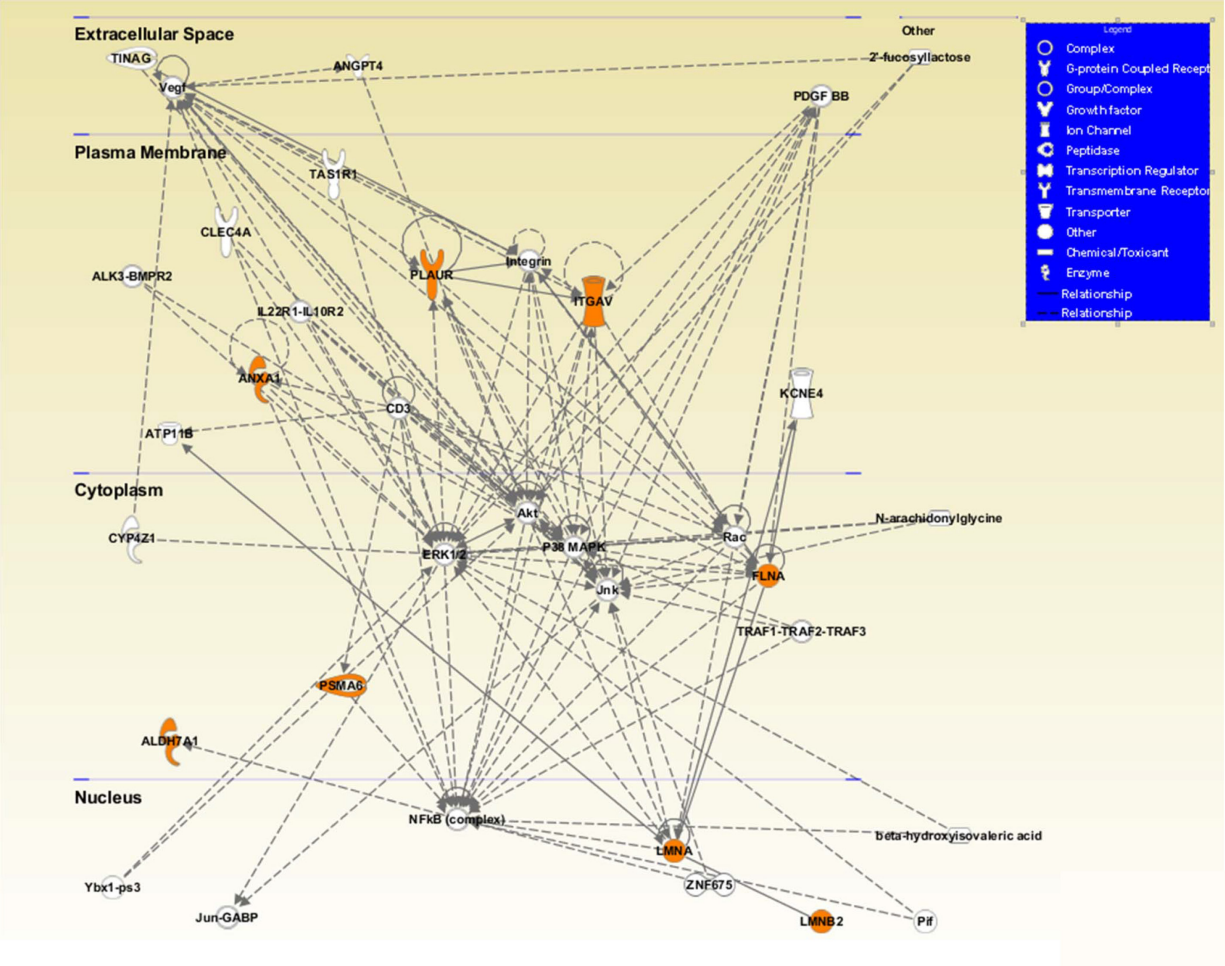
Visual representation of the principal network generated by Ingenuity Pathway Analysis (IPA) The network included 6 of the 7 validated proteins with direct and indirect relations (ANXA1, FLMNA, PSMA6, ALDH7A1, LMNA, LMNB2) connected with both αv (ITGAV) and uPAR (PLAUR) proteins and localized to a specific cell compartment (all colored in orange). Network proteins are visualized by proper symbols, which specify the functional nature of protein. Each node represents a protein and its direct (represented by solid lines) and indirect (represented by dotted lines) association with other proteins. Proteins with no background color were undetected in the study but have been inserted by IPA to produce a highly connected network.
